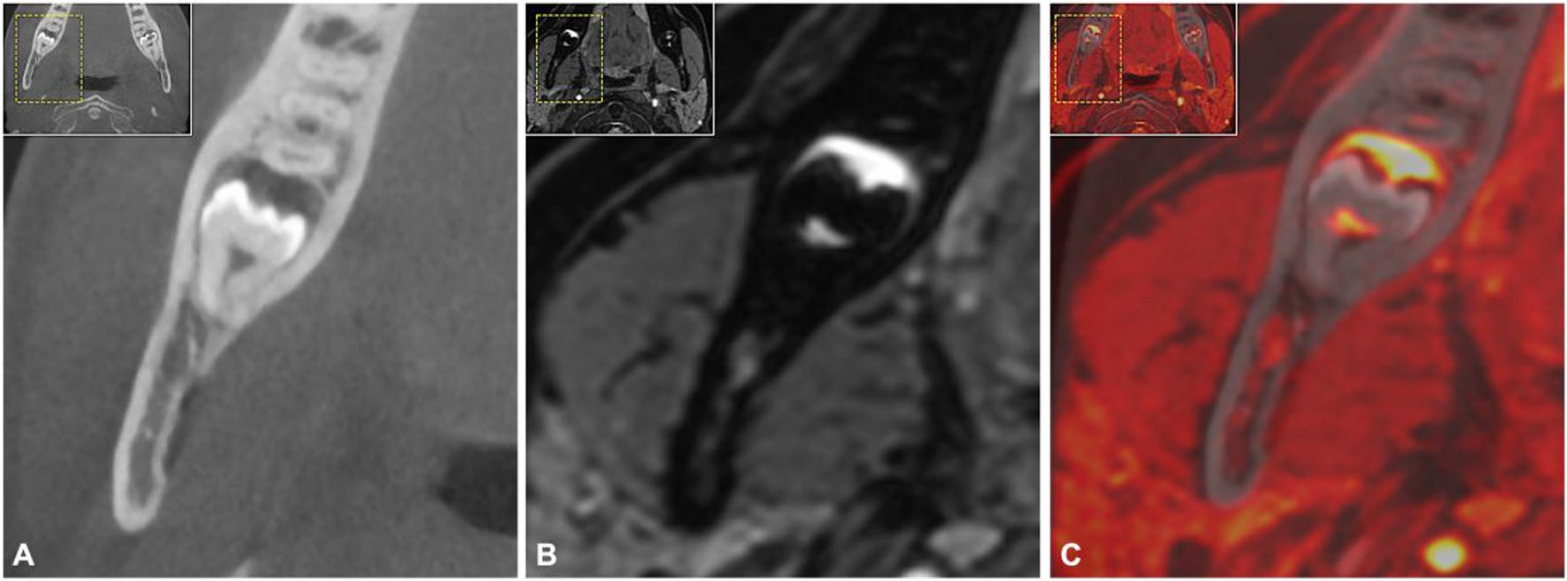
a CBCT, b MRI, and c fused CBCT/MRI imaging of the positional relationship between the mandibular canal, respectively, inferior alveolar nerve and the mandibular third molar. This figure displays the advantages and disadvantages of each imaging modality and their respective limitations. Depending on the imaging modality, relevant information is provided, such as direct visualization of the inferior alveolar nerve (b) or only the osseous boundaries of the MC (a). In addition, an inflammatory process most consistent with a follicular cyst is also visualized
